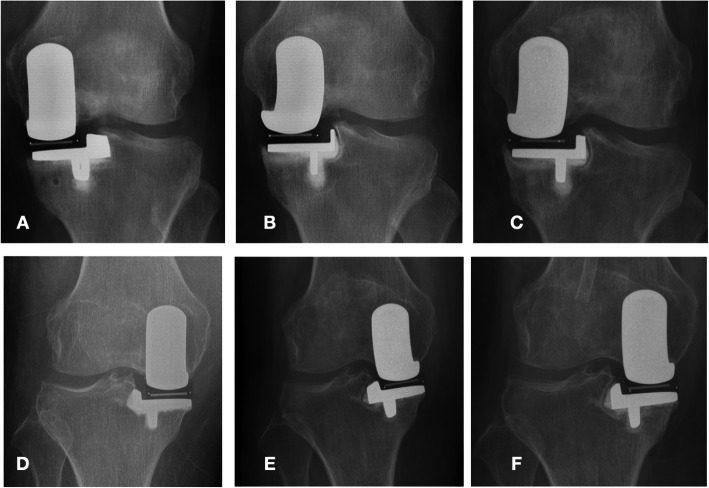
shows 2 patients developed radiolucent line after surgery (6 months after surgery, 1 year after surgery), both the 2 patients did not have pain or other symptoms. a, b and c are the X-rays of the patient 3 months after surgery, 1 year after surgery and 2 years after surgery. d, e and f are the X-rays of another patient 2 weeks after surgery, 6 months after surgery and 1 year after surgery
